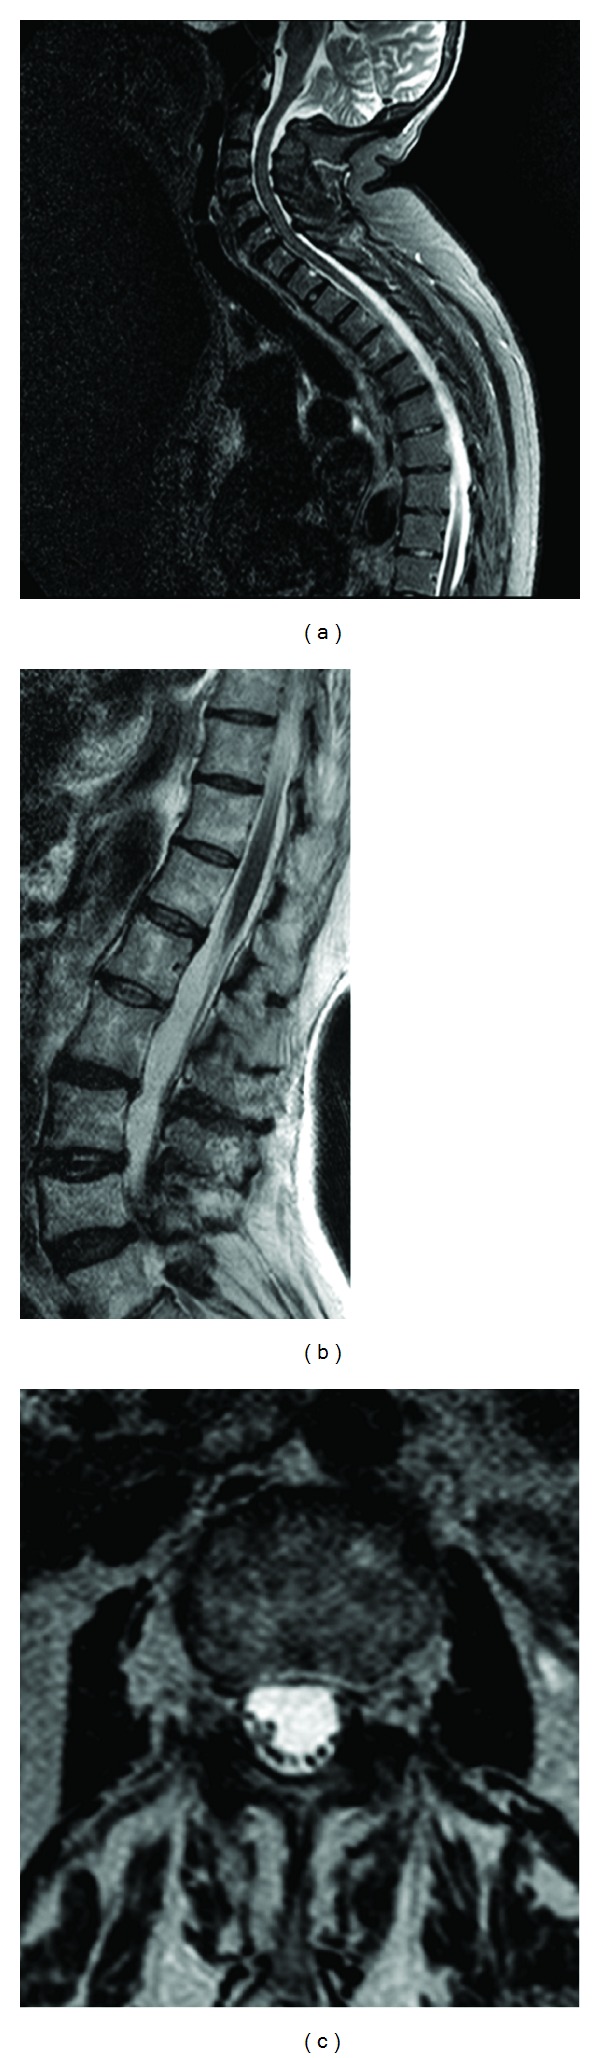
Hypointensities around the brainstem and all along the spinal cord are depicted in both sagittal MRI STIR-adquired images ((a) and (b)). The thecal *cul-de-sac* is occupied by iron-containing debris (b). There are also signs of arachnoiditis such as lumbar nerve root clumping ((b) and (c)).
